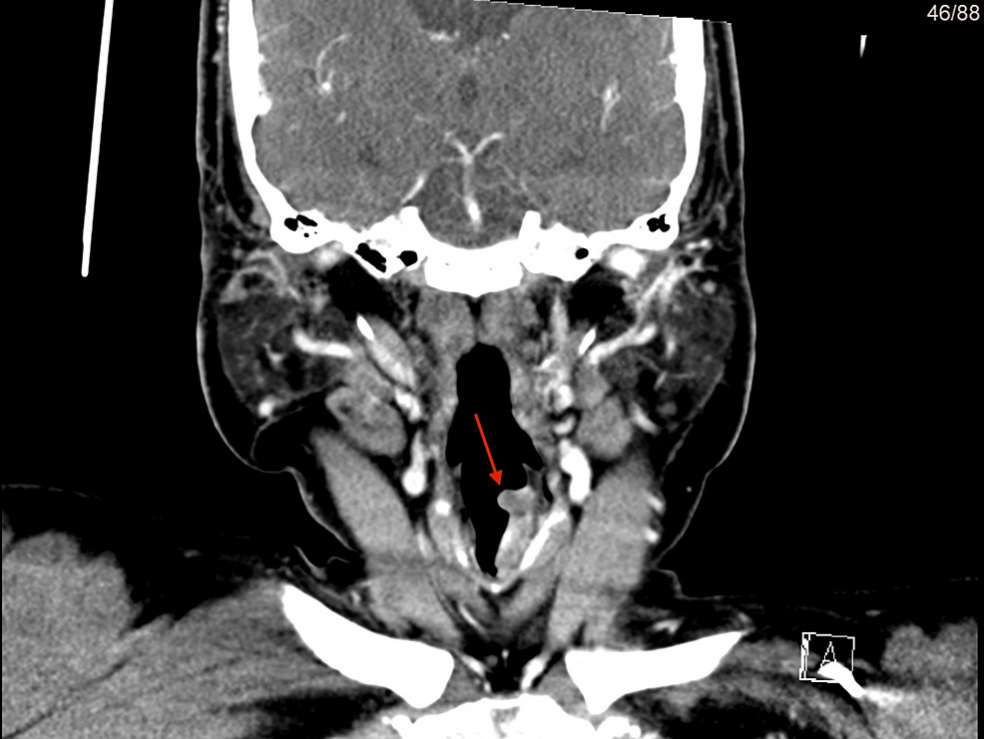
CT scan in coronal view of the mass shown by the red arrow.
